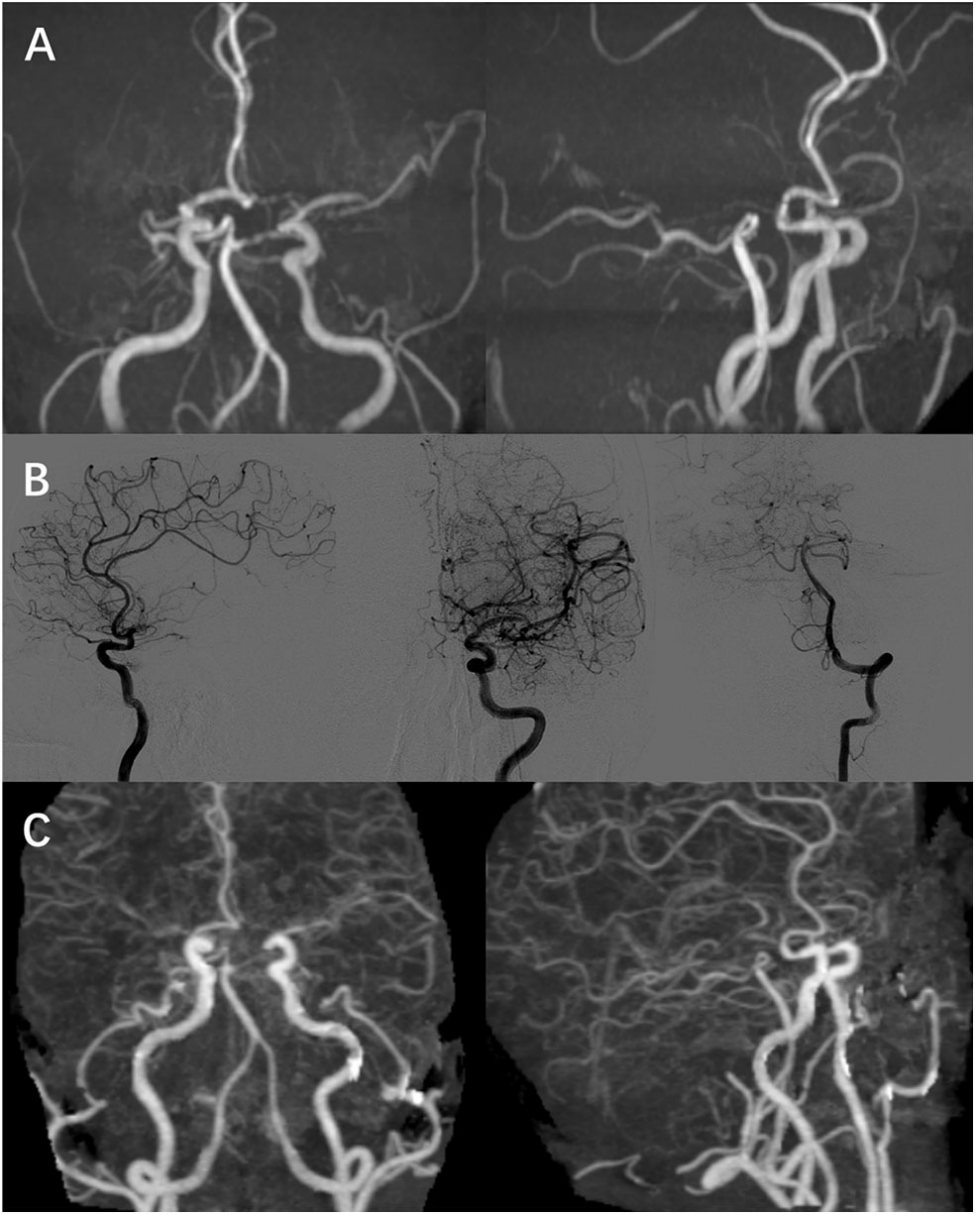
Image result of cerebral vessels. (A,B) MRA and DSA showed that right MCA was occulted as well as bilateral terminal ICA, and the left MCA, ACA, and PCA were narrow, which was accompanied with “puff-of-smoke” vessels. (C) CTA showed “puff-of-smoke” vessels and meningeal branch neovascularization after the 2-stage bilateral STA bypass surgery. ICA, internal carotid artery; MCA, middle cerebral arteries; ACA, anterior cerebral arteries; CTA, computed tomography angiography.
